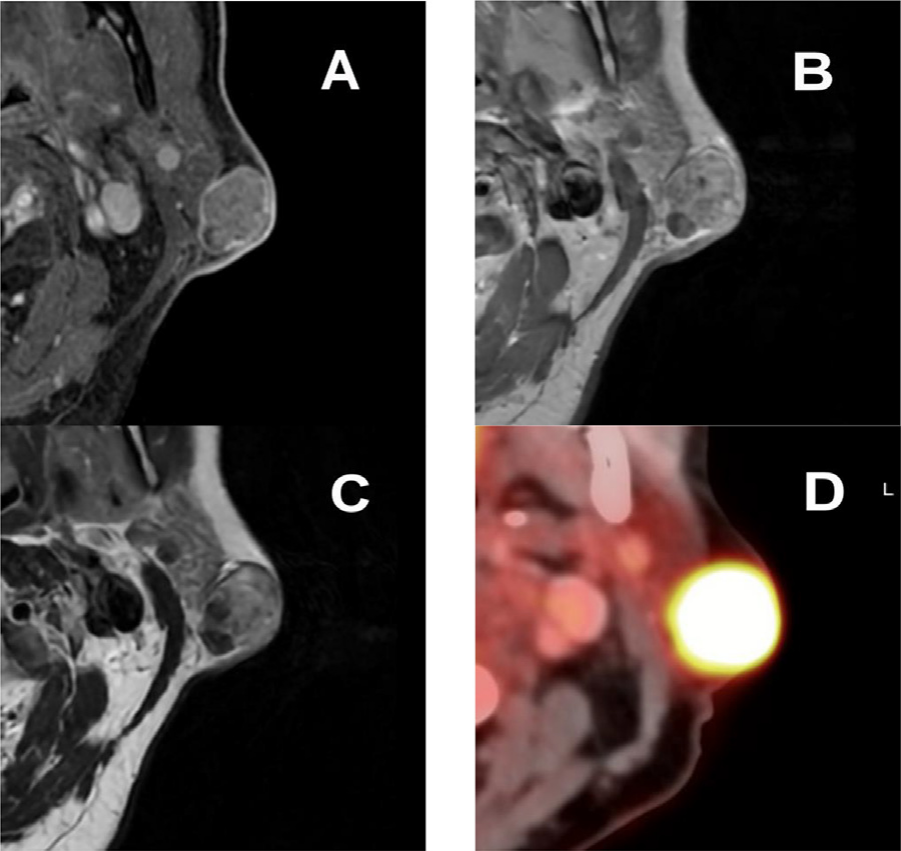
(A) Gadolinium-enhanced fat saturation T1 weighted images MRI with marked enhancement of the lesion. (B) Gadolinium-enhanced T1 weighted images MRI with heterogenous enhancement of the lesion. C) T2-weighted images MRI showing a heterogenous subcutaneous mass in contact with the parotid gland. D) FDG PET-CT showing a hypermetabolic mass in the left parotid region with a SUV of 18.
